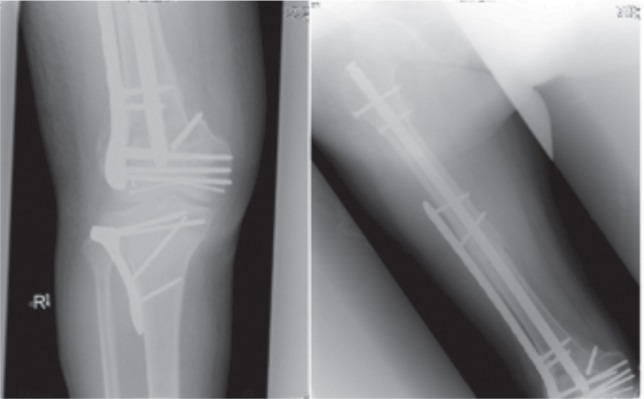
Radiographs post 2nd injury definitive fixation.
